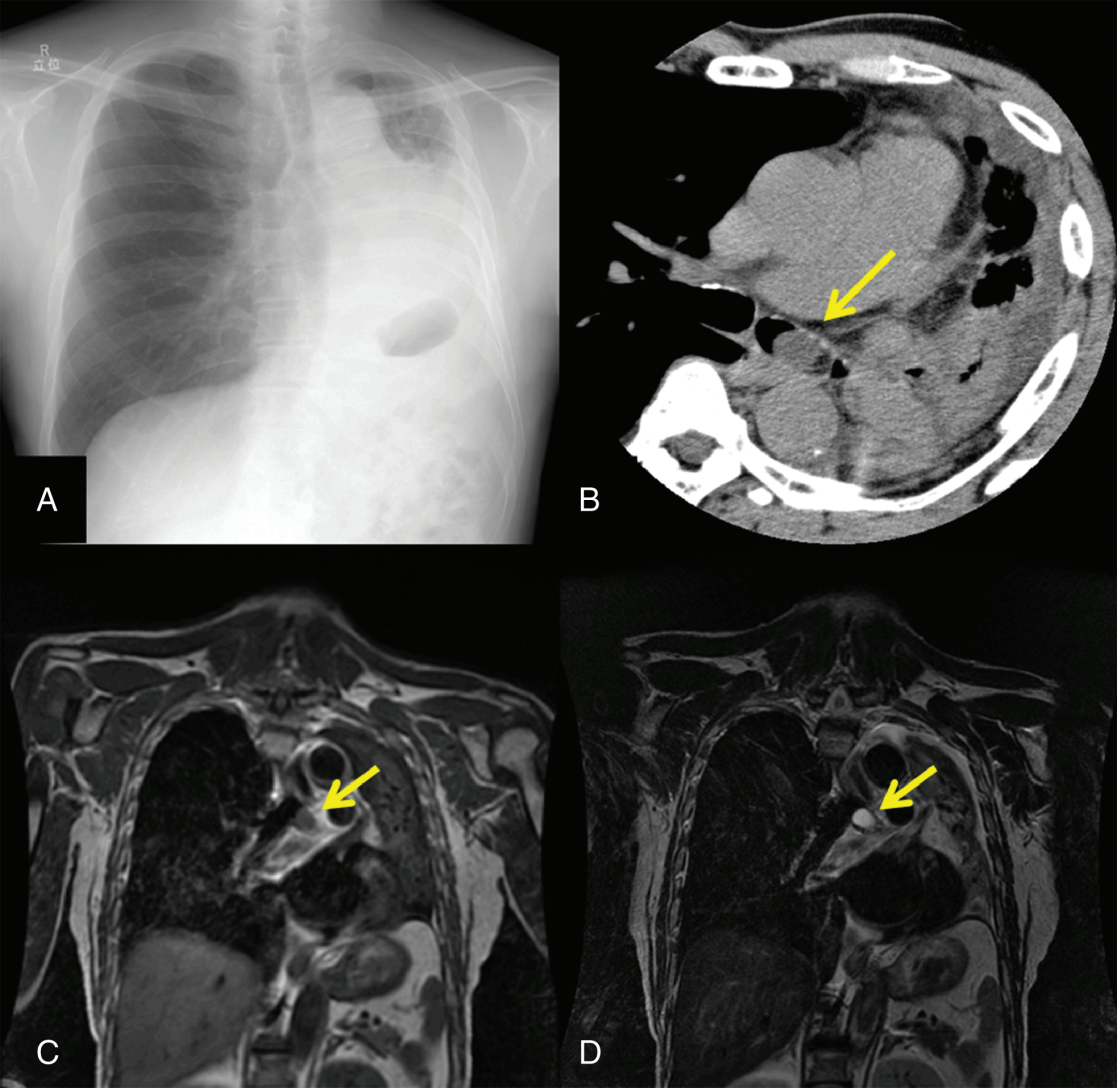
(A) The chest radiography findings led to a diagnosis of obstructive pneumonia and atelectasis. (B) Computed tomography revealed a mass in the left main bronchus. Magnetic resonance imaging revealed water signal intensity. (C) T1‐weighted imaging. (D) T2‐weighted imaging.
